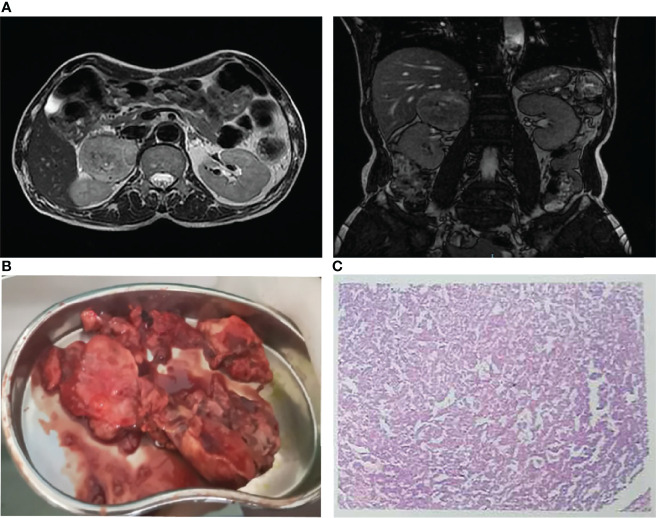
(A) Magnetic resonance imaging demonstrated a 68 x 61 mm mass in the right adrenal gland. (B) Right adrenalectomy was performed to remove a huge pheochromocytoma. (C) Pathological examination showed pheochromocytoma.
